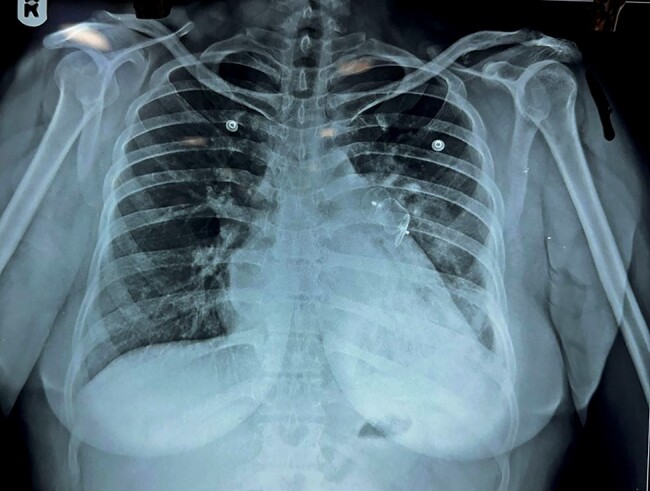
Post-intervention chest X-ray showing the vascular plug *in situ*.
